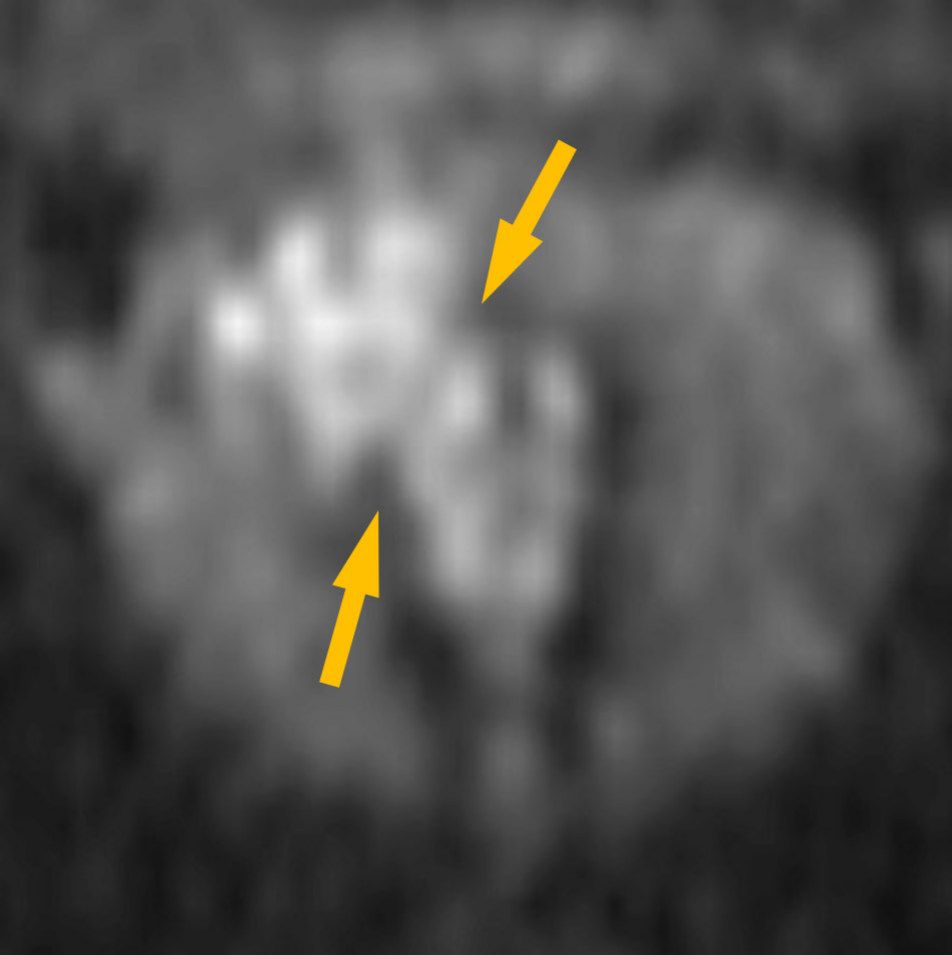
MPR coronal image with the prostatic urethra as the axis on DWI. The continuity between the lesion in the prostatic urethra and the nodule in the right transition zone became clearer (→).
